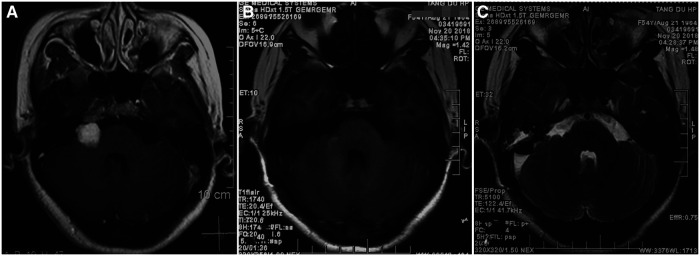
MRI features of the patient before and after craniotomy. (A) Axial T1-weighted MRI with contrast showing a 1.5 cm*1.2 cm*1.3 cm right-sided vestibular schwannoma before craniotomy surgery; (B) Postoperative axial T1-weighted MRI with contrast revealing total resection of tumor; (C) Postoperative axial T2-weighted MRI showing no ischemic lesion in the right AICA territory.
